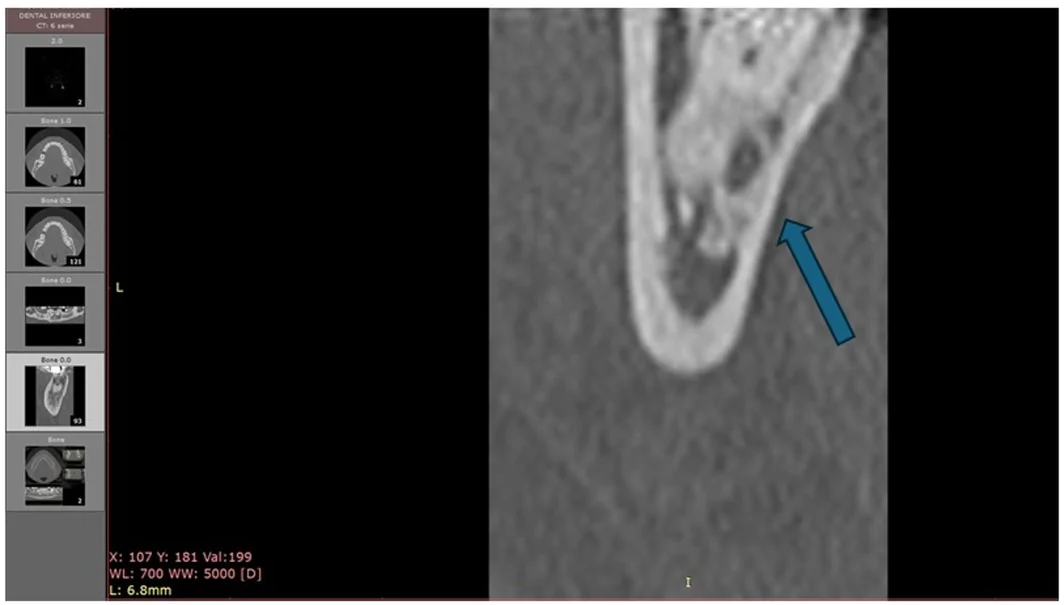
Coronal CBCT section showing the dental apices extending beyond the inferior alveolar nerve, which is located lingually. The roots are fused at the apical level.
